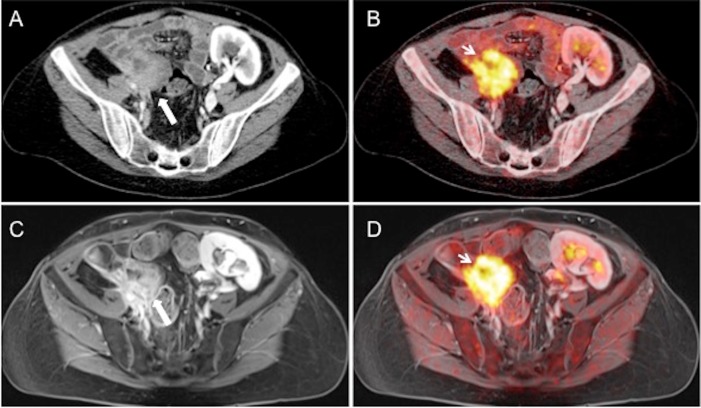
A 46-year-old patient with recurrent cervical cancer. Tumor lesion originating from right ovary with contact to the small bowel is hardly detectable in CT (A). The identical lesion shows a slightly higher lesion contrast in the post-contrast VIBE sequence in MRI (C). In fused PET/CT (B) and PET/MR (D) images the lesion can be equally clearly identified as a metastasis.
